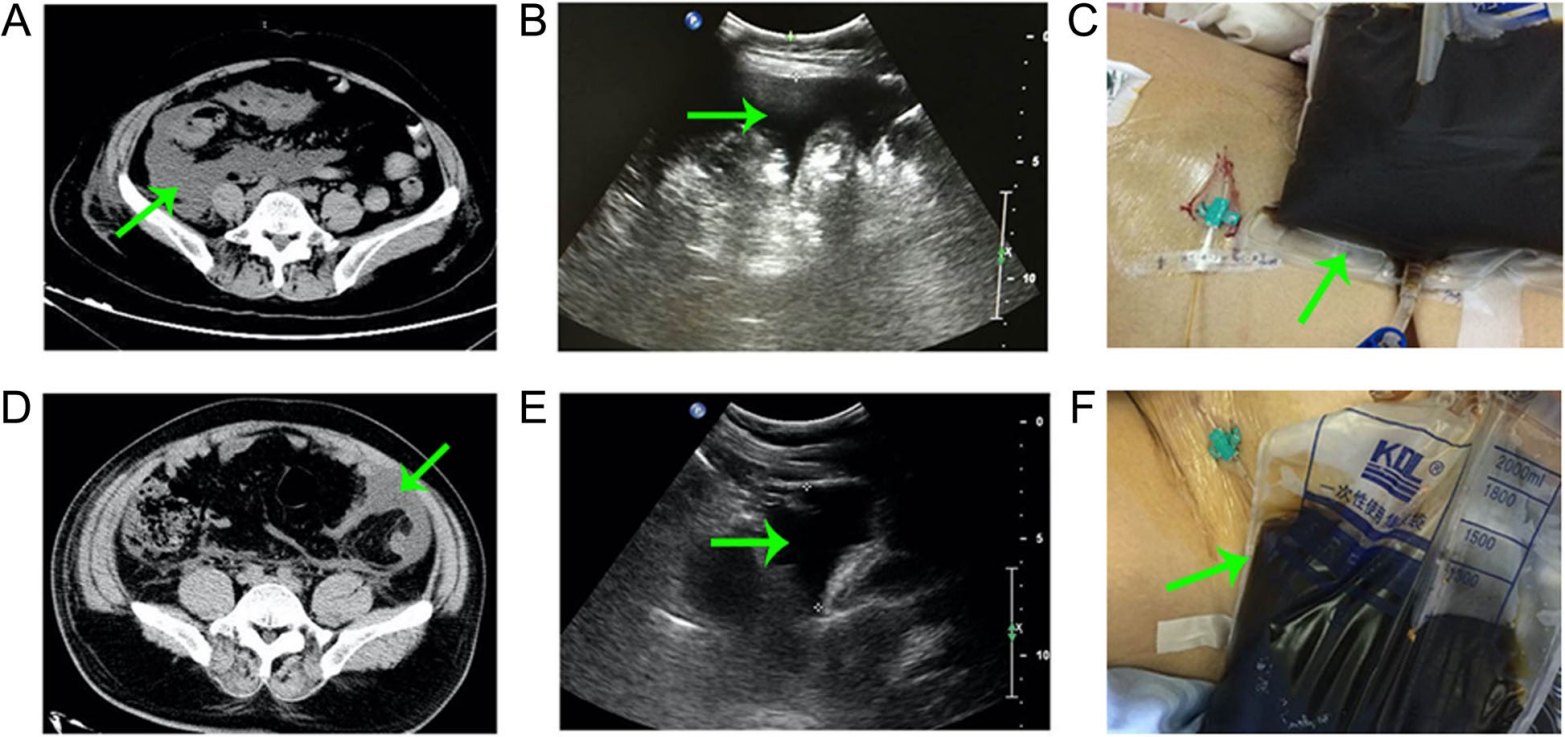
Pelvic ascites images obtained from two patients via computed tomography scan, ultrasound and macroscopy. A–C Computed tomography scan, ultrasound results and macroscopic pelvic ascites of one patient. D–F Computed tomography scan, ultrasound results and macroscopic pelvic ascites of another patient
